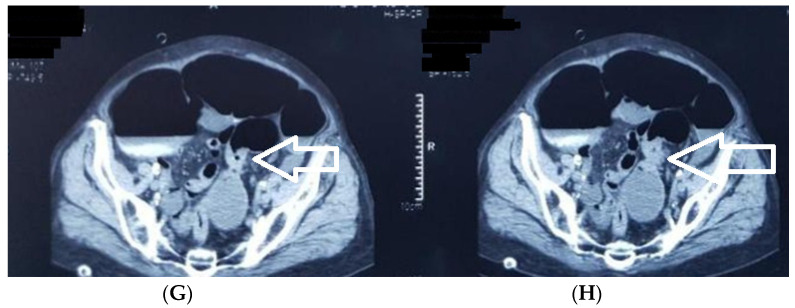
Abdominal and pelvic CT scans of colorectal tumors. (A) A 77-year-old male patient with a stenotic tumor on the transverse colon and a distended proximal colon, with air-fluid level, axial section (tumor highlighted with a white arrow); (B) The patient mentioned above, axial section—distended cecum with a high risk of perforation (white arrow); (C) The patient mentioned above, coronal section, tumor highlighted with a white arrow; (D) Another 77-year-old male patient with a sigmoid tumor with invasion in the urinary bladder, highlighted with a white arrow, axial section; (E) The same patient, coronal section, distension of the colon, sigmoid tumor highlighted with a white arrow; (F) The same patient, sagittal section, distension of the colon, sigmoid tumor highlighted with a white arrow; (G) A patient with a stenotic tumor of the colorectal junction (white arrow), with upper distension of the digestive tract; (H) The same patient, axial section; the CT scan reveals a distended colon and the tumor highlighted with a white arrow.
